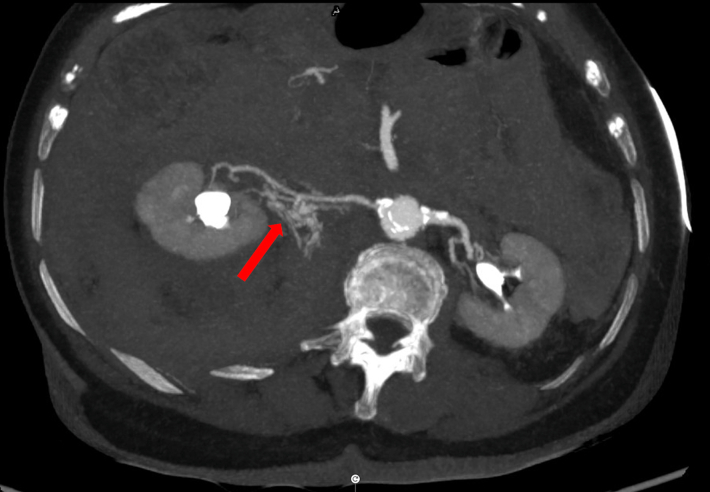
Cross-Sectional Computed Tomography Image of the Abdomen Showing Contrast Extravasation From the Right Renal Artery (Red Arrow)
